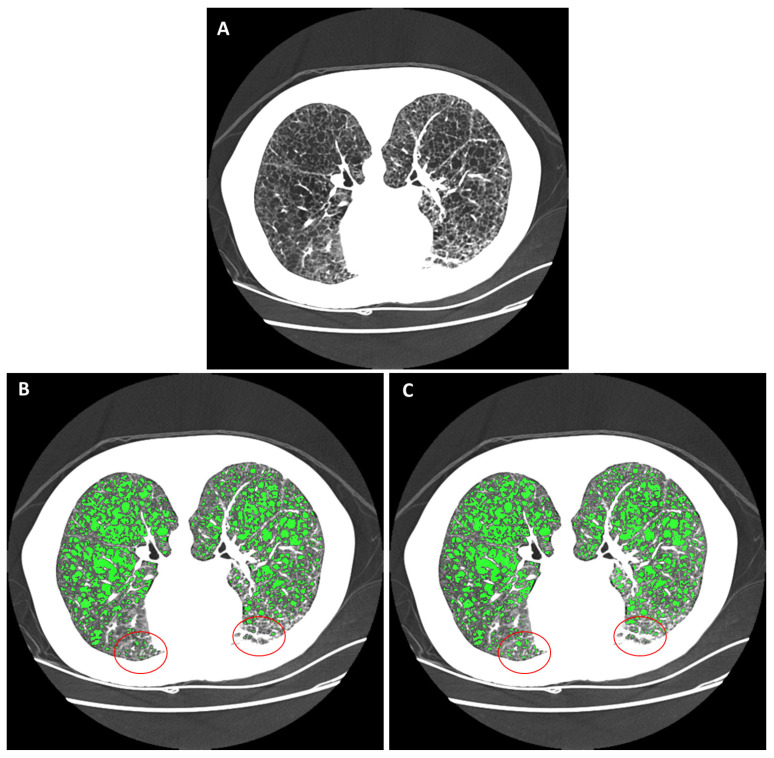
An example of within-section variability of the radiodensity of the pulmonary parenchyma and its impact on cyst segmentation. (A) An HRCT section of a LAM patient lying in the prone orientation is displayed in the grayscale range of −1100 HU to −500 HU. The anterior portion of the lungs was enhanced due to positional atelectasis. (B) The gold-standard semi-automated segmentation by a trained operator, who set a global threshold for cystic areas to −930 HU. Cystic areas are highlighted in green. The anterior region of the lungs was under-segmented due to the elevated parenchymal radiodensity there (highlighted by the red circles). (C) The automated cyst segmentation. The automated method accounted for regional variations of radiodensity, which mitigated the problem of under-segmentation in the anterior region.
